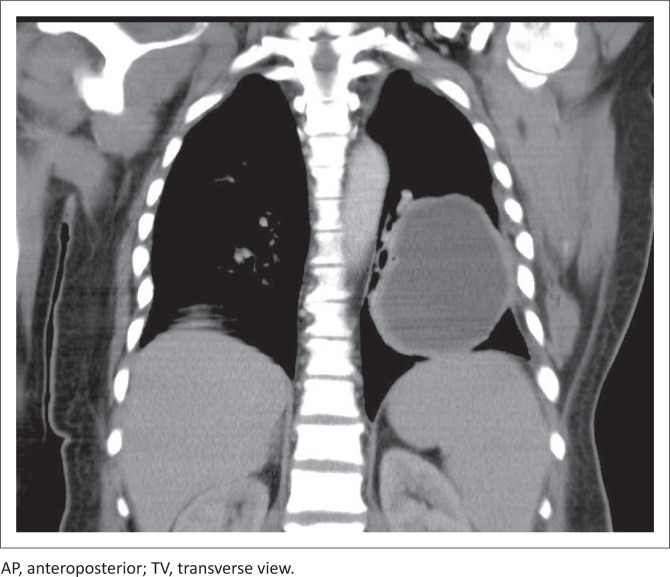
Computed tomography scan of the chest showing cystic lesion measuring 91 mm AP × 90 mm TV in left lower lobe.
